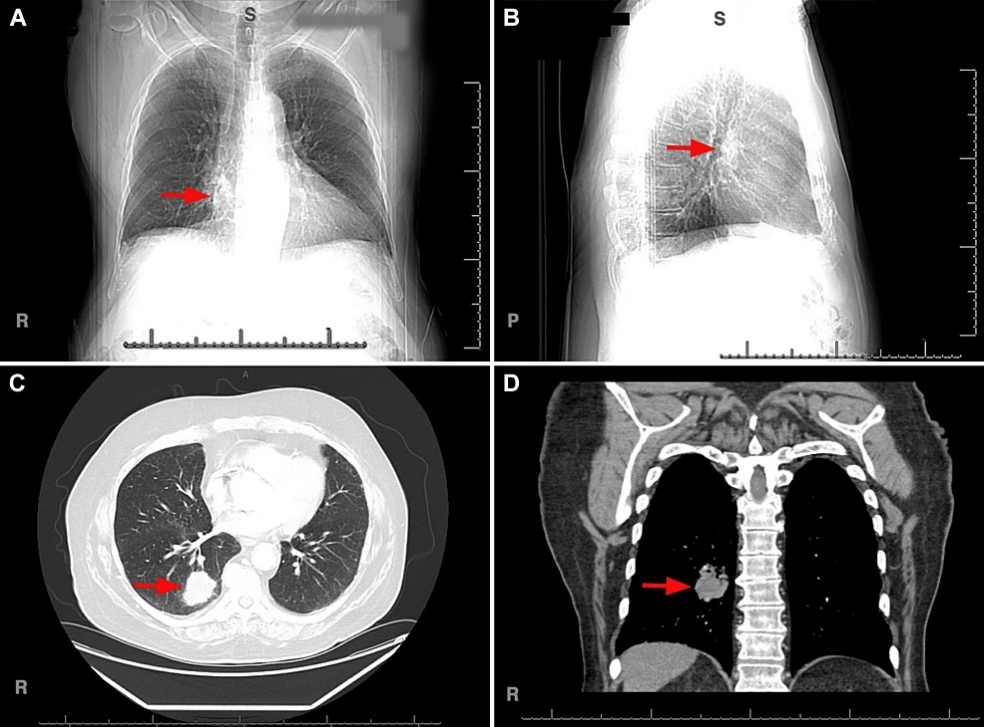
Chest x-ray anterior and posterior view, red arrows indicating the lesion (A and B). CT scan with contrast, red arrows indicating the lesion (C and D).
